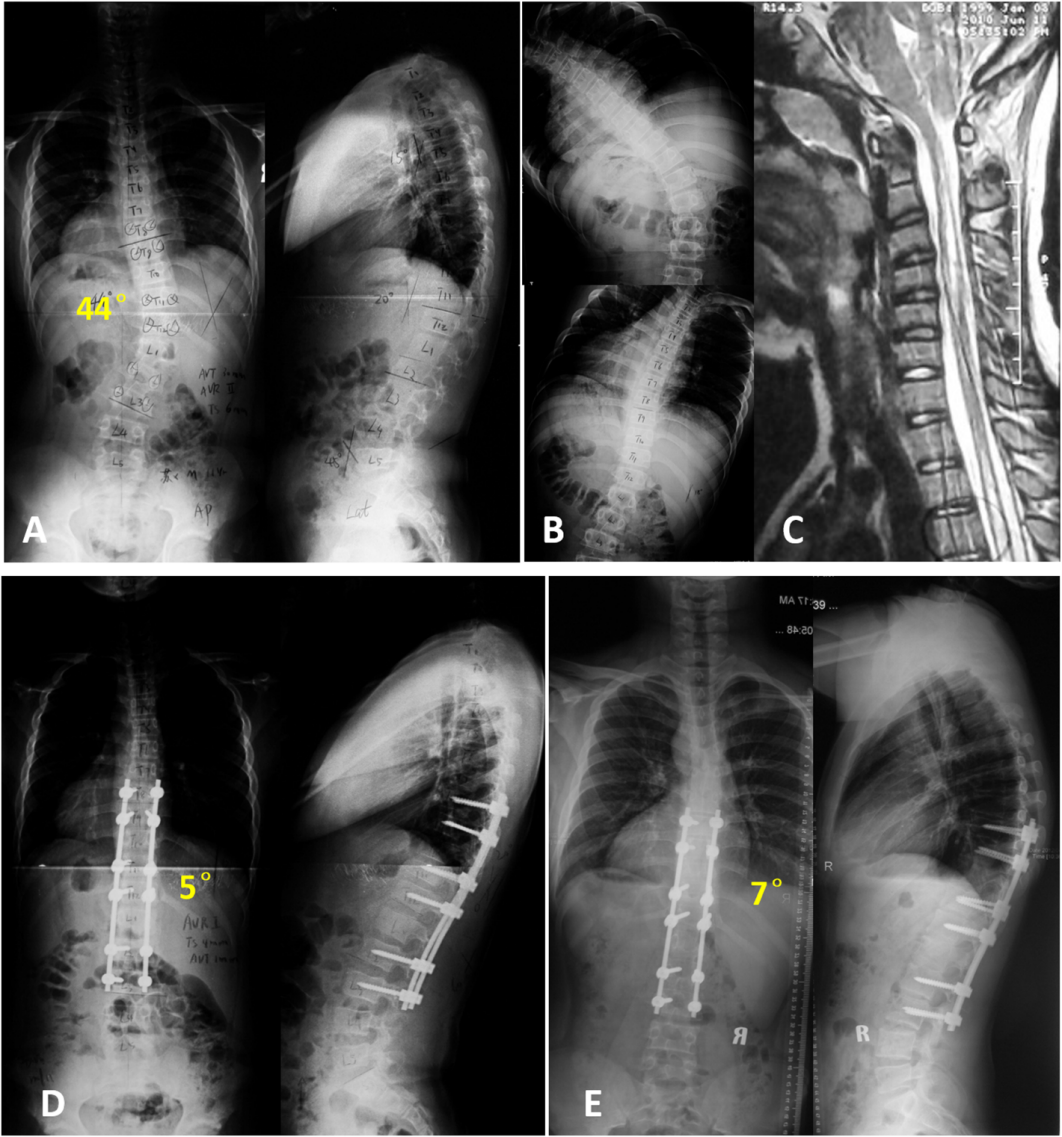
12-year-old boy with a major thoracolumbar curve (44°) secondary to syringomyelia (a). Preoperative bending radiograph shows the high flexibility of major thoracolumbar curve (b). Sagittal views of the syrinx located at cervical and thoracic region (slender type) (c). Posterior selective thoracolumbar fusion from T8 to L3 achieved effective correction of scoliosis (d). Radiographs made at five years follow-up (e)
